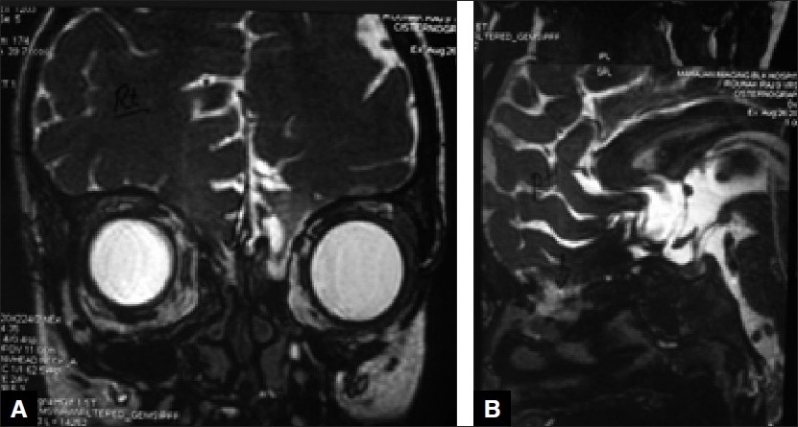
MRI cisternography coronal (A) and sagittal (B) views showing the bilateral cribriform plate defects
